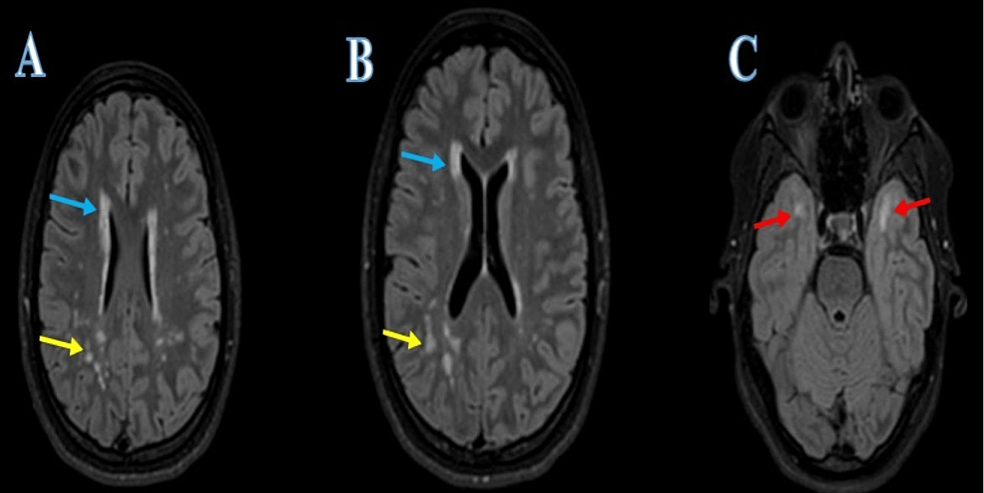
MRI image of patient's head A. Extensive white matter abnormality with multiple foci of increased fluid-attenuated inversion recovery (FLAIR) and T2 signal evident within the periventricular white matter and subcortical white matter in the frontal (blue arrows) band parietal lobes (yellow arrows). B. Confluent areas of the abnormal signal were noted in the periventricular right frontal lobe (blue arrows) and parieto-occipital lobe (yellow arrows). C. FLAIR hyperintensity is evident in the subcortical white matter of the anterior temporal lobes (red arrows), a typical location of CADASIL.
